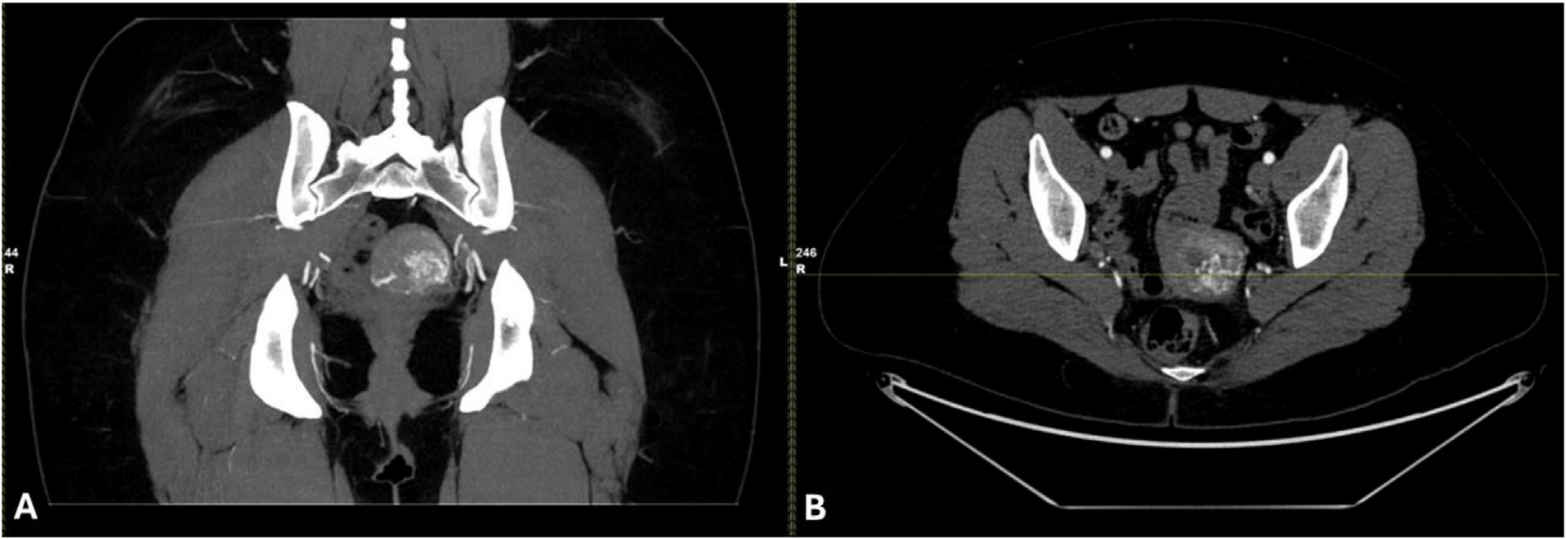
CTA in coronal (A) and axial (B) views. Clear visualization and comparisons with the prior MRI pelvis favor towards an AVM due to the early filling of the cluster of vessels that appeared to be predominantly located in the myometrium. Views also suggest that both left, and right uterine arteries are involved. Note: CTA, Computer tomography angiography; MRI, Magnetic resonance imaging; AVM, Arteriovenous malformation.
